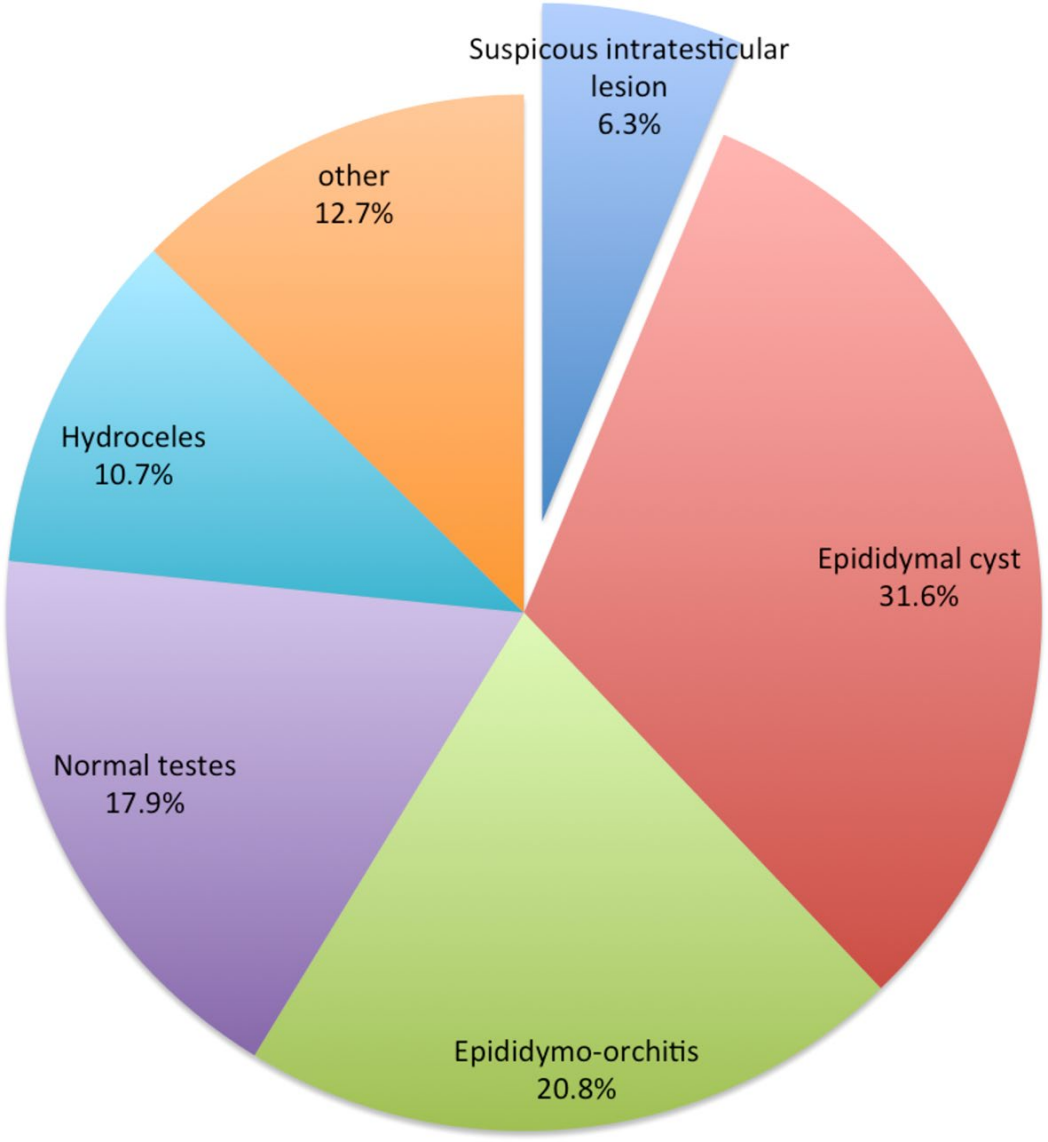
A chart describing all testicular ultrasound scans results
